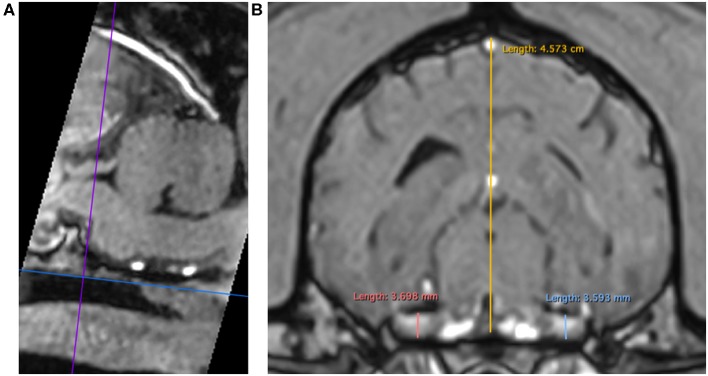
MPR of a 3DT1+C sequence of the brain of a Labrador Retriever (TR = 20.2 ms, TE = 10.3 ms, ST = 1 mm). Saggital reconstruction (A). The dorsal axis (blue line) is adjusted parallel to the basisphenoid bone to ensure the transverse axis (purple line) is perpendicular. Transverse reconstruction (B). Trigeminal nerves are identified coursing horizontally just caudal to the foramen ovale. Caliper measurements for HBR calculation: TTH left (light blue line), TTH right (coral pink line), and BH (yellow line). MPR, multiplanar reconstruction; 3DT1+C, three-dimensional T1-weighted post-contrast; TR, repetition time; TE, echo time; ST, slice thickness; TTH, trigeminal nerve transverse height; BH, brain height; HBR, height-to-brain ratio.
